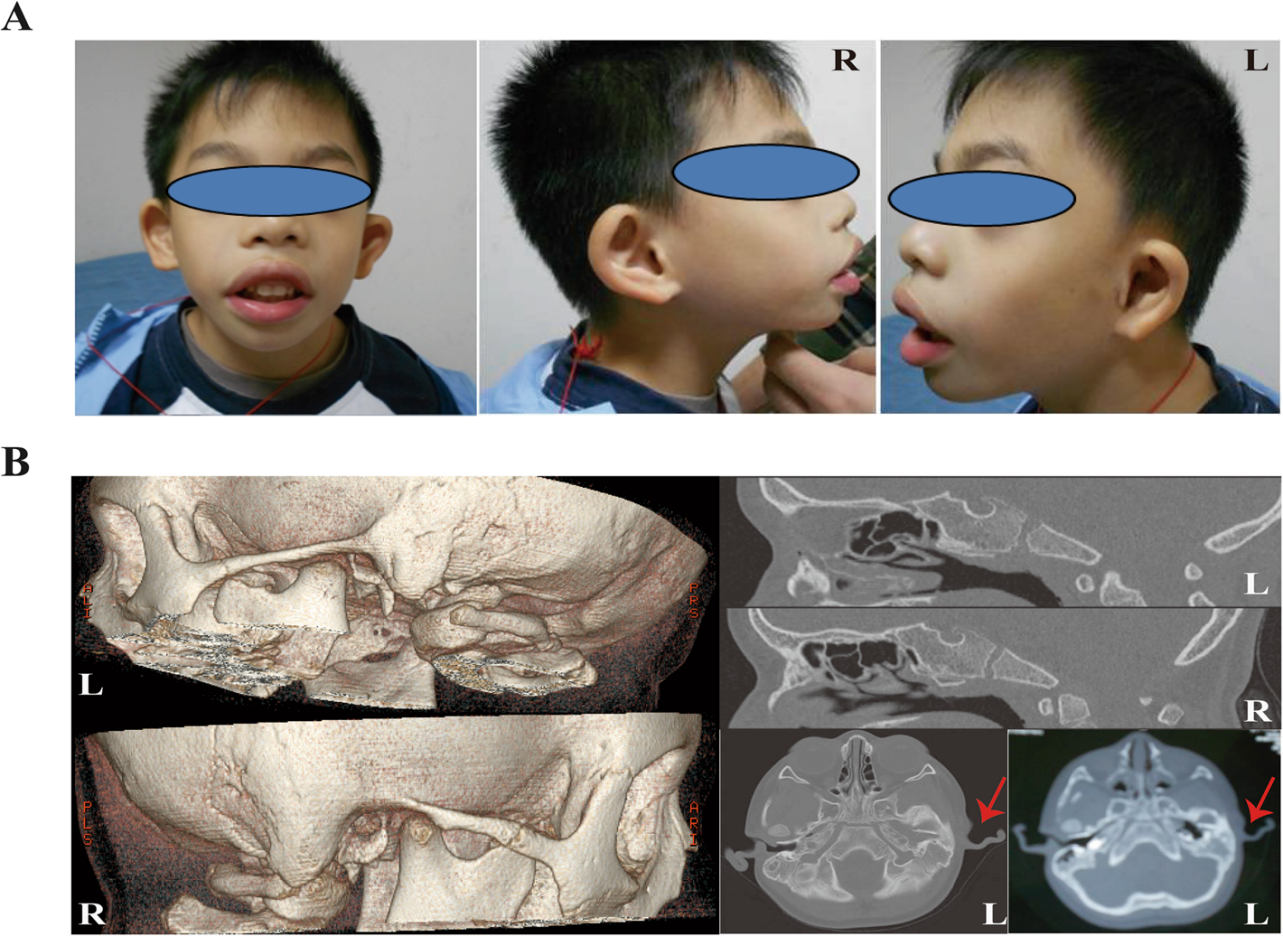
Clinical presentation of the proband. a Microcephaly, abnormal external ear, and mandibular hypoplasia features of the patient at 57 months of age. b Malformed structures of the external and middle ear on the left head and temporal bones via CT scan at 37 months of age; soft tissue could be seen in the left tympanic cavity. R, right; L, left
